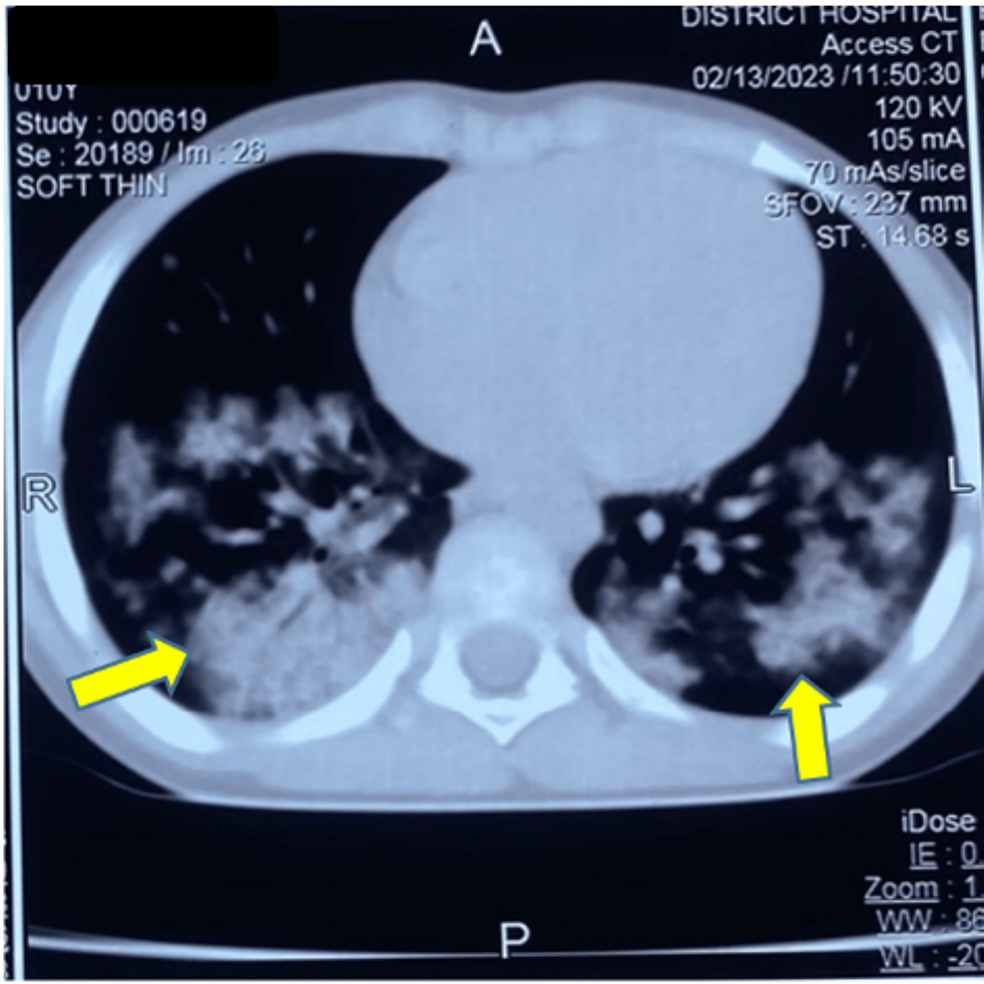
Computed tomography of the chest showing bilateral patchy hyperdense lesions in lung parenchyma.
